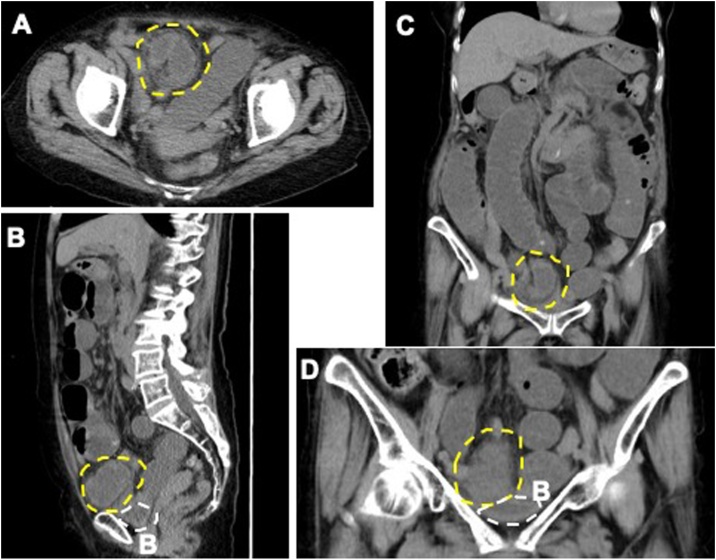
CT scan revealing a sac-like mass involving a dilated bowel loop (yellow dotted lines) descending anteriorly and laterally with respect to the bladder (B/white dotted line). Axial (A), sagittal (B) and coronal (C,D) sections.
